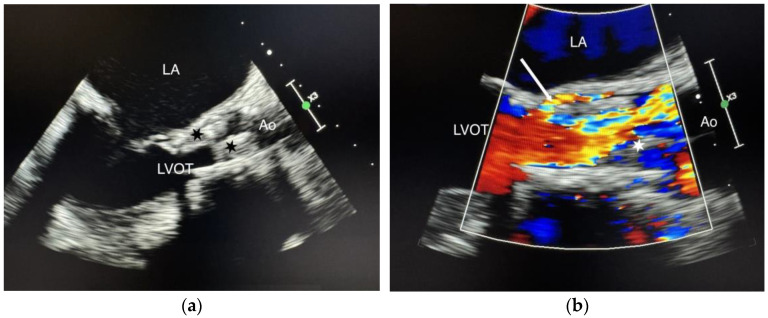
(a) Standard TEE showing the vegetations. Cusp enlargement and vegetations (black stars); (b) Color Doppler TEE demonstrating paravalvular regurgitation. Vegetation (white star), paravalvular regurgitation (white arrow), LA (left atrium), LVOT (left ventricular outflow tract), Ao (aorta).
